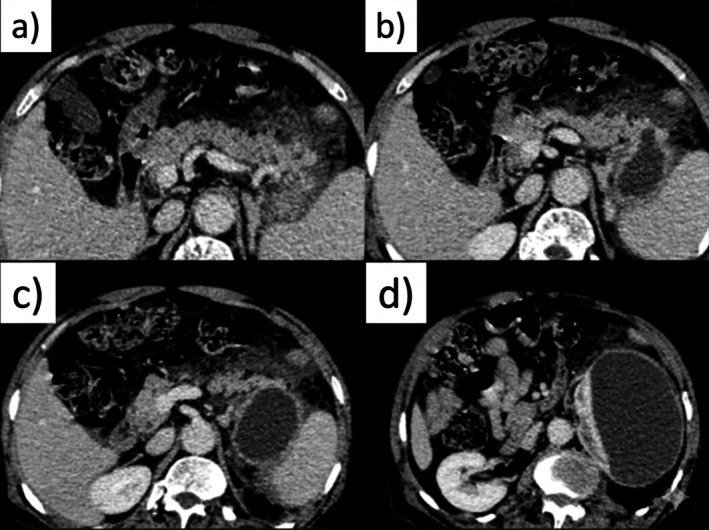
Contrast‐enhanced CT scan of the abdomen in axial view demonstrating a peripancreatic fluid collection (a–d). (a–d) Serial axial CT images show a well‐defined, low‐attenuation fluid collection in the lesser sac, located posterior to the stomach and anterior to the left kidney. The lesion is consistent with a peripancreatic fluid collection, with mild mass effect on surrounding structures and without evidence of pancreatic necrosis.
